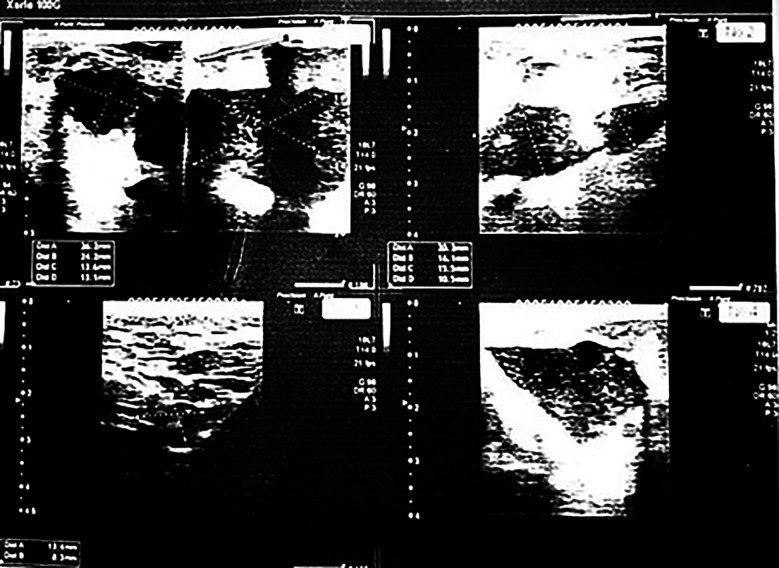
Ultrasound of the right breast demonstrating a well-defined hypoechoic lesion with internal echoes in the retro-areolar region, consistent with a localized abscess cavity.
